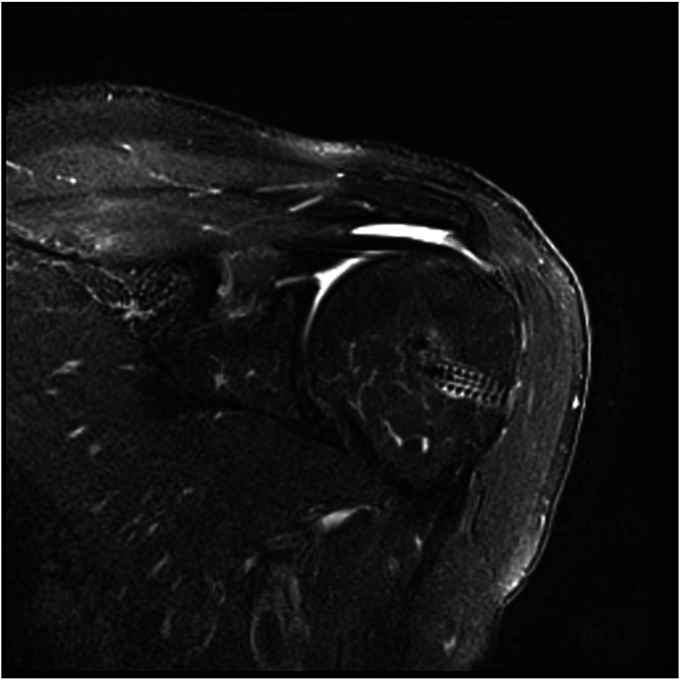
Healed massive cuff repair with thinning of the tendon (Sugaya grade III) of patient 8. MRI performed 10 months after surgery.
